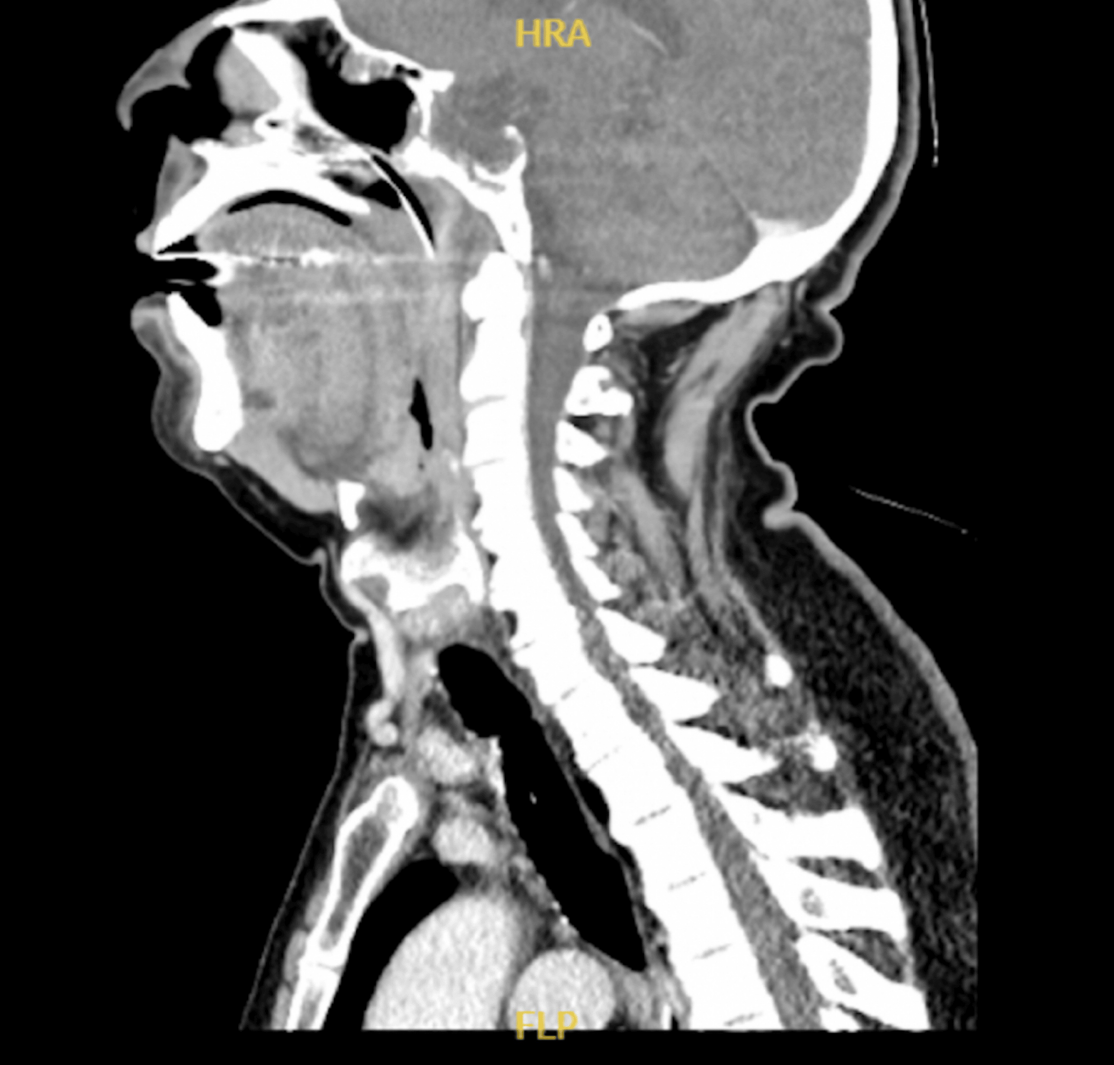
Sagittal ceck CT with IV contrast Inflammatory process of the right side of the oropharynx and right palatine tonsil, with no evidence of localized fluid collections or abscesses
